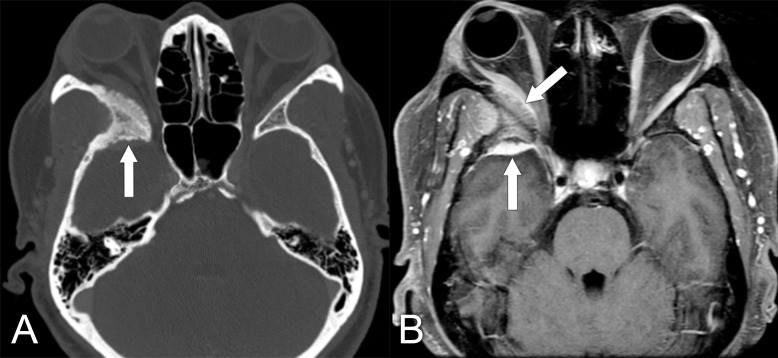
Imaging findings in a patient who presented with slowly progressive proptosis of the right eye. (A) Axial CT, bone window, shows hyperostosis of the lateral right orbital wall and sphenoid bone (arrow). (B) Axial T1 postcontrast MRI with fat suppression shows an enhancing mass involving the right sphenoid bone, posterior orbit, and middle cranial fossa (arrows). The diagnosis was confirmed to be sphenoid wing meningioma.
